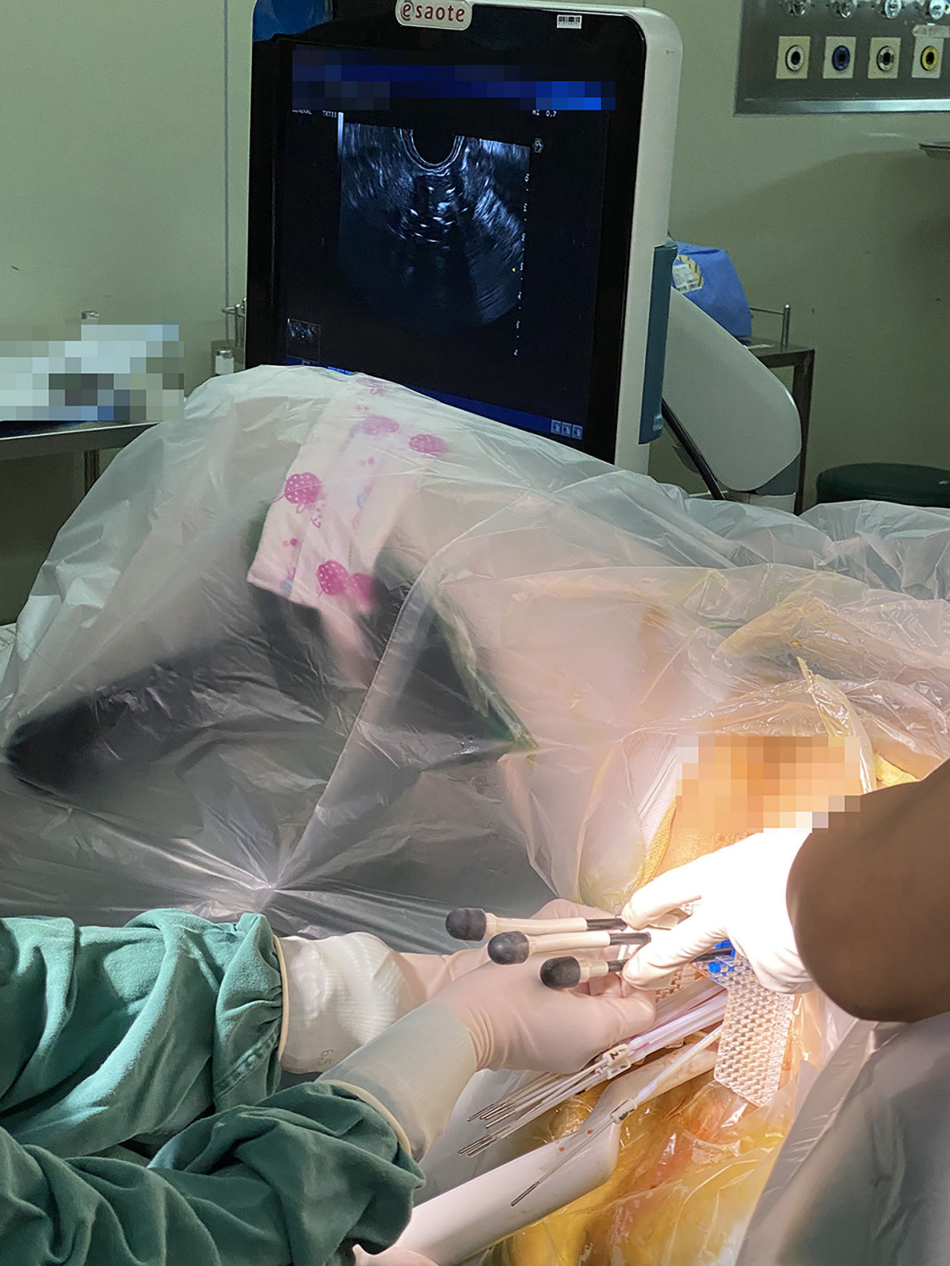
Use of hybrid intracavitary and interstitial (IC/IS) brachytherapy with assistant of real-time transrectal ultrasound. All the implants were MR compatible.
